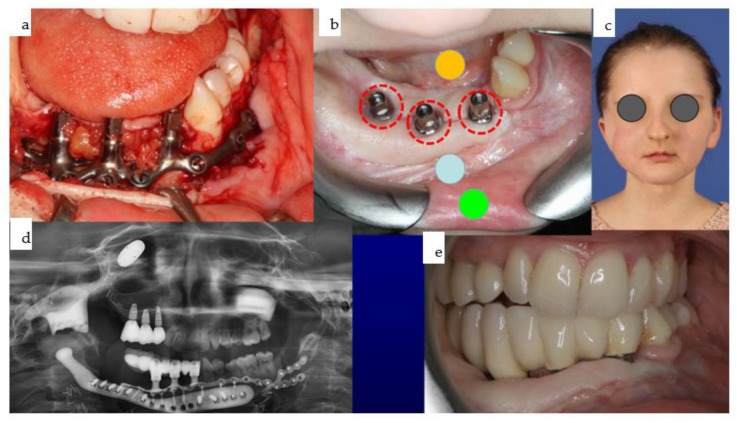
(a–e): Different views of the IPS Implants^®^ Preprosthetic during (a) and three months after (b) insertion are presented; the red dotted circles around the posts reveal healthy tissues (b). The yellow (floor of the mouth), light blue (vestibule), and green (inner lining of the lip) spots demonstrate the successful subunit separation due to the additional microvascular radial forearm flap, which was placed ahead of bimaxillary dental rehabilitation. The clinical appearance is depicted in (c); the orthopantomogram summarizes all biomaterials and implants placed into the patient over many years, including the gold weight implant for the right upper eyelid, patient-specific PEEK implant (non-radio-opaque) for the right temporal fossa (only fixation screws are visible), right total joint replacement, IPS Implants^®^ Preprosthetic with mounted final superstructure, and the conventional three bone-level tapered dental implants with crowns in the right maxilla (d). An intraoral view matching the clinical picture (c) is shown in (e) with the dental arches in occlusion. Mild right mandibular sagging led to occlusal correction over time.
